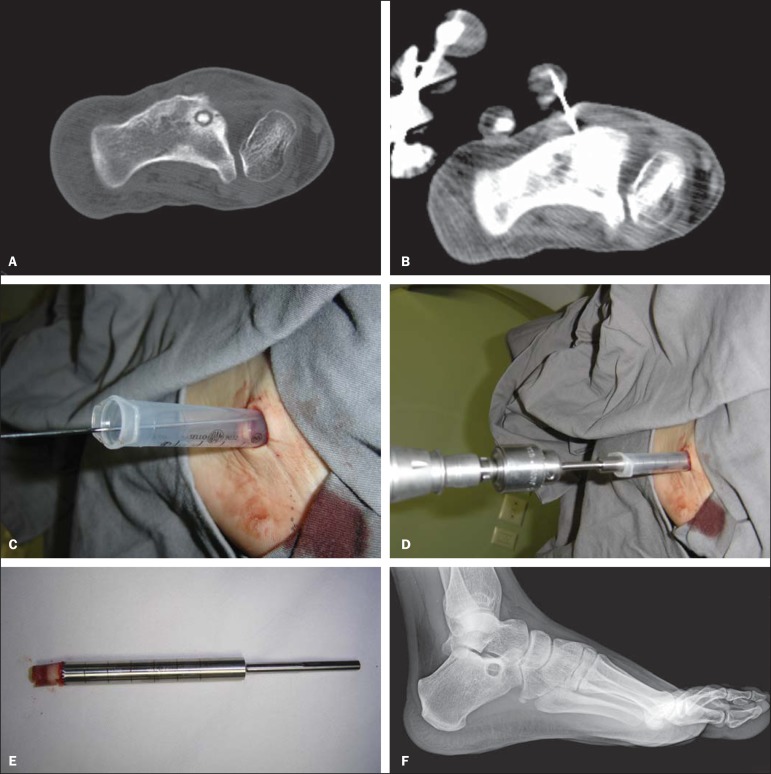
A: A well-defined round nidus is visible in the calcaneus CT image.
